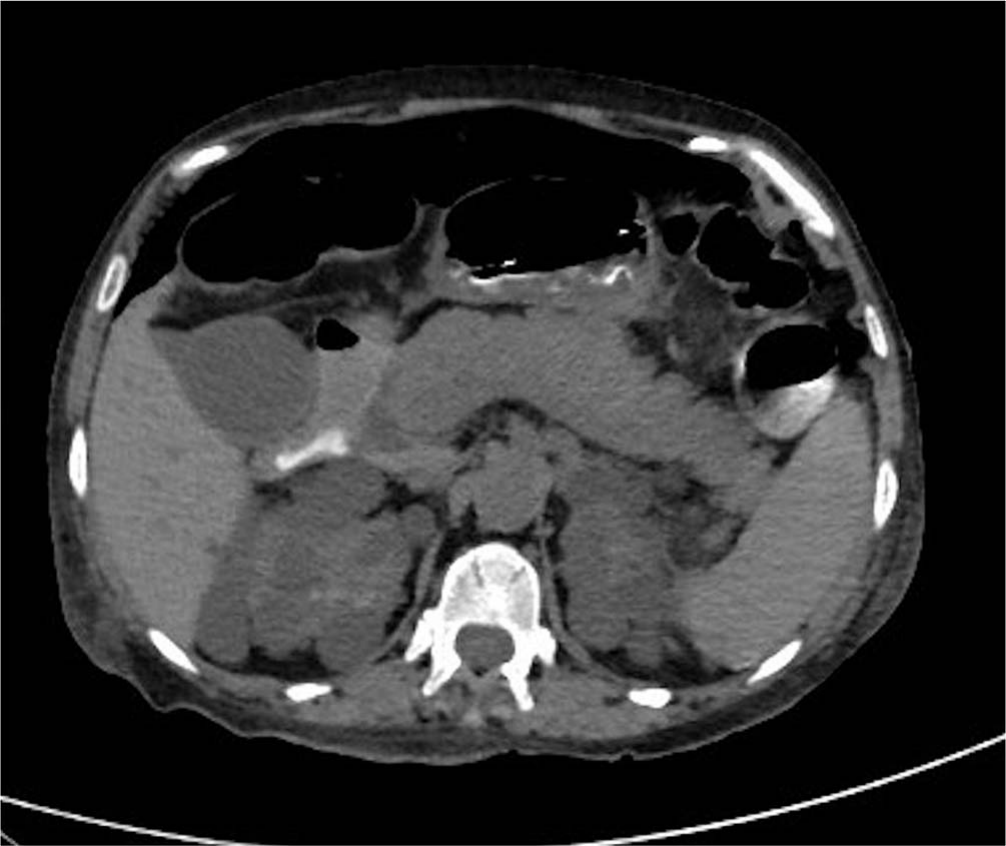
CT scan of abdomen and pelvis: pneumoperitoneum and polycystic disease in the kidneys, liver, and pancreases.
